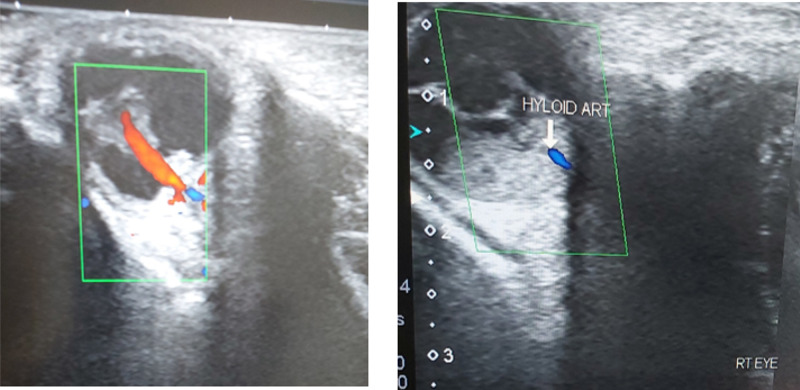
Doppler ultrasonography showing the presence of the hyaloid vasculature
